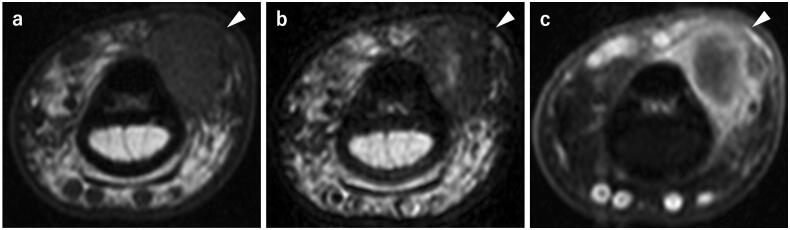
Preoperative magnetic resonance imaging. a T1WI; A well-defined mass, 1 cm in diameter, showing iso signal intensity. b T2WI; A mass showing low signal intensity with partial internal high signal intensity. c Fat-suppressed T1WI-gadlinium showing enhancement on the margin of the mass.
